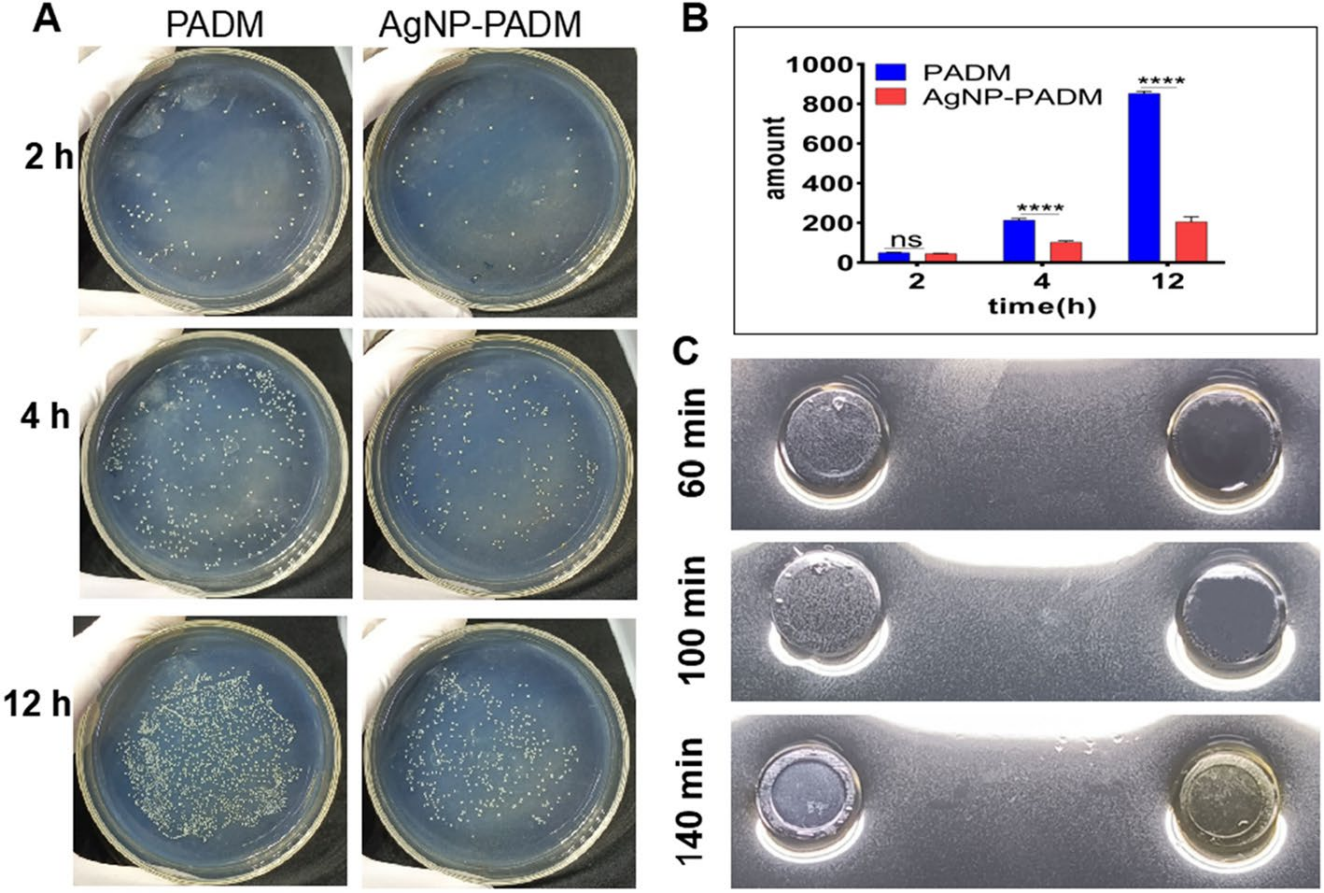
A, B PADM and AgNP–PADM planktonic bactericidal effect measured using dilution-plate method. C Bactericidal effect of AgNP solution released during ultrasonic shocking
